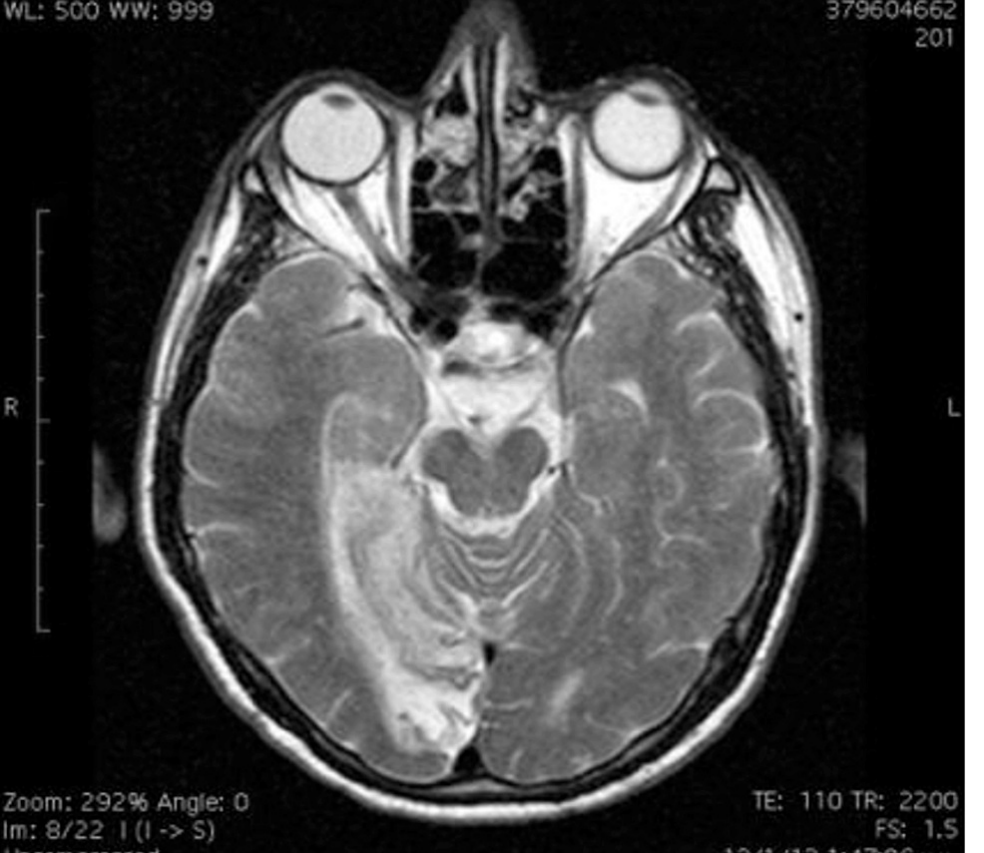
Cerebral MRI showing ischemic infarction in the region of the right occipital lobe, the cognate temporal lobe, the corporis callosi, and the ventral thalamus.
